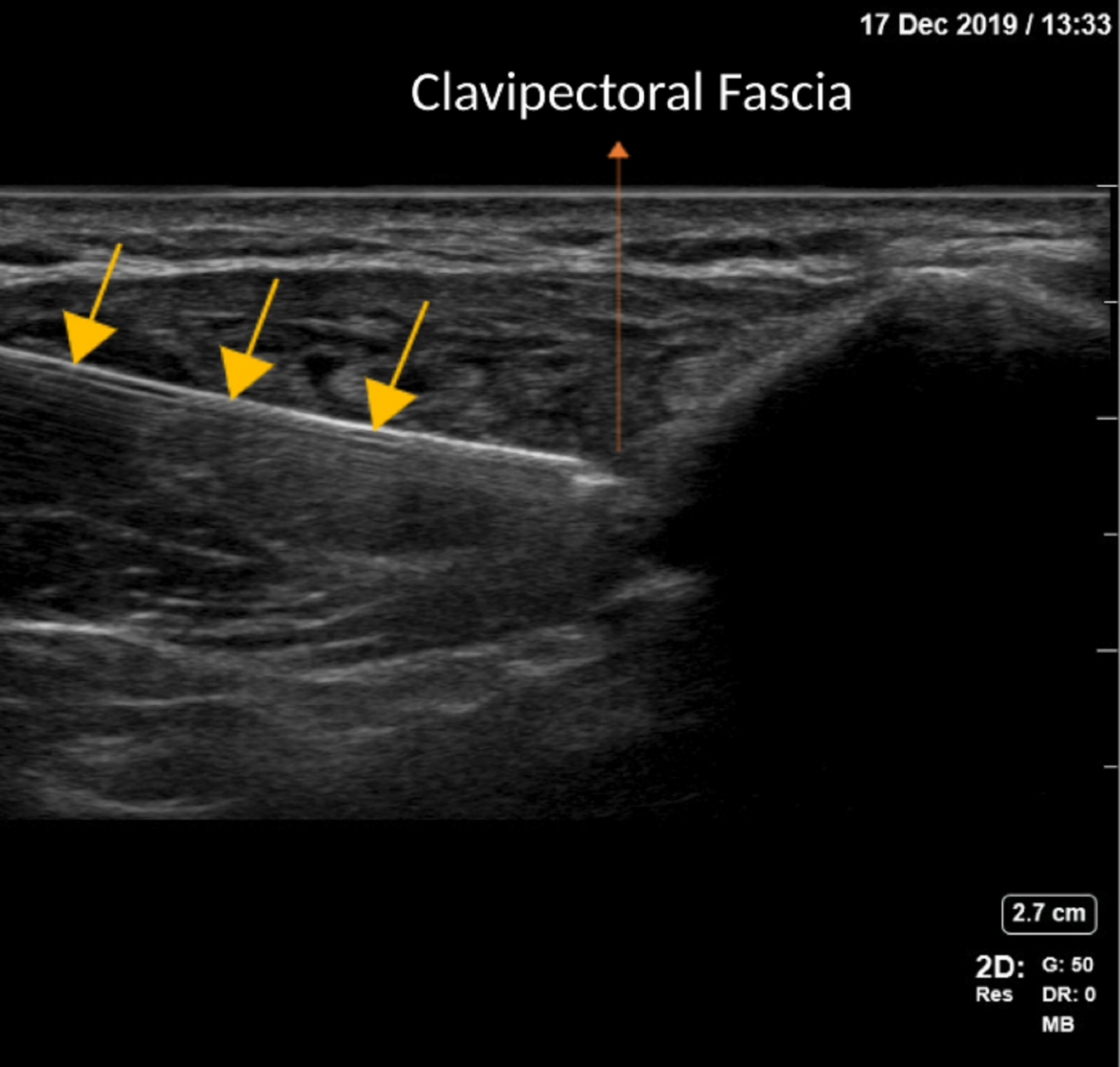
Ultrasound image of in-plane needle technique for CPB CPB: clavipectoral fascial plane block
